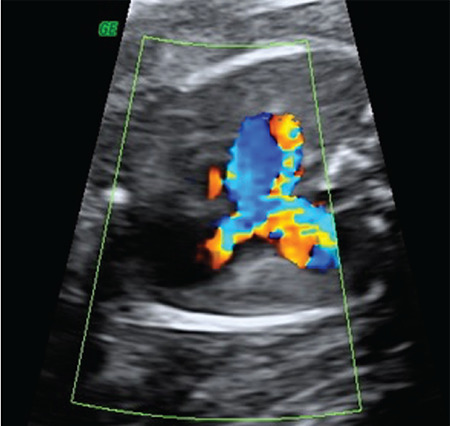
Increased peak flow velocity through the pulmonary valve annulus and a retrograde diastolic flow originating from the pulmonary valve annulus was revealed by color Doppler ultrasound
